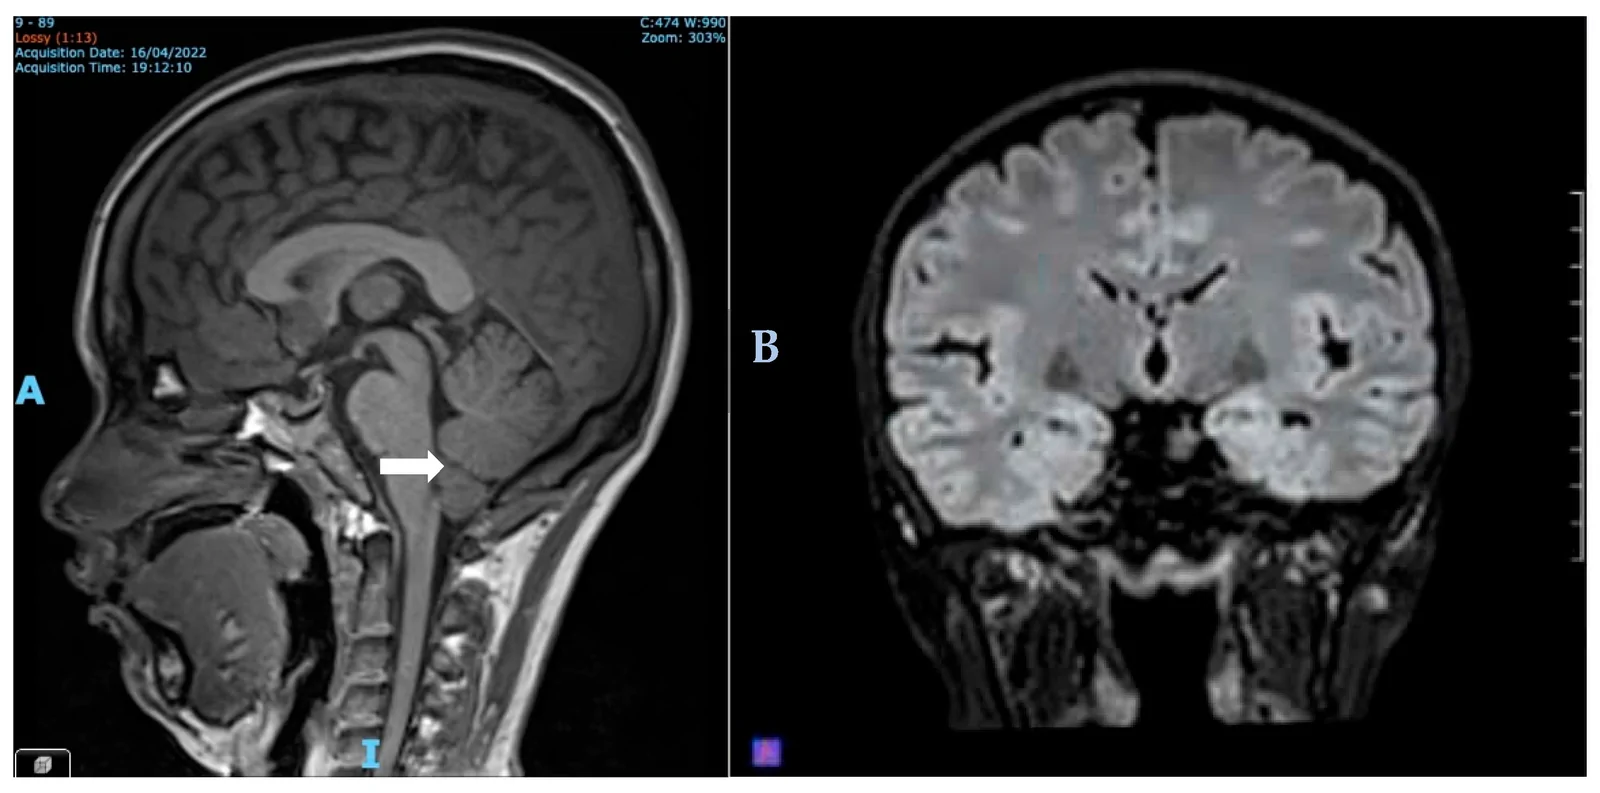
Brain MRI showing (A) low-lying cerebellar tonsils below the foramen magnum (white arrow) in Case 1, and (B) normal findings in Case 2.
